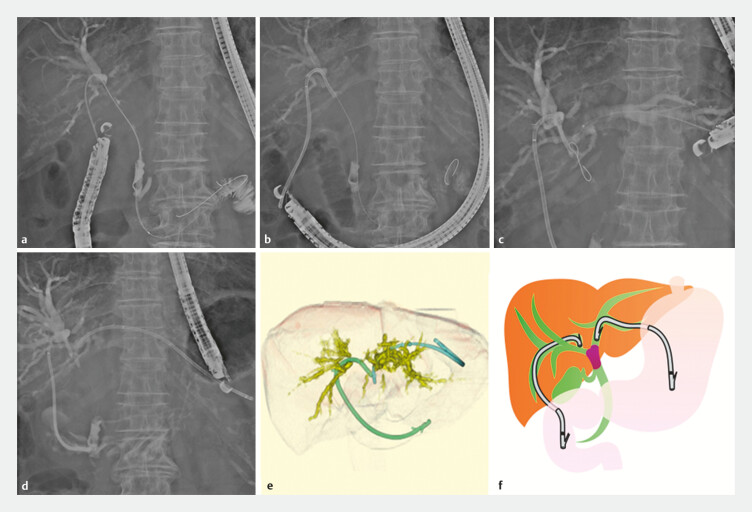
Case of endoscopic ultrasound-guided hepaticoduodenostomy and hepaticogastrostomy (EUS-HDGS) with a plastic stent (PS).
